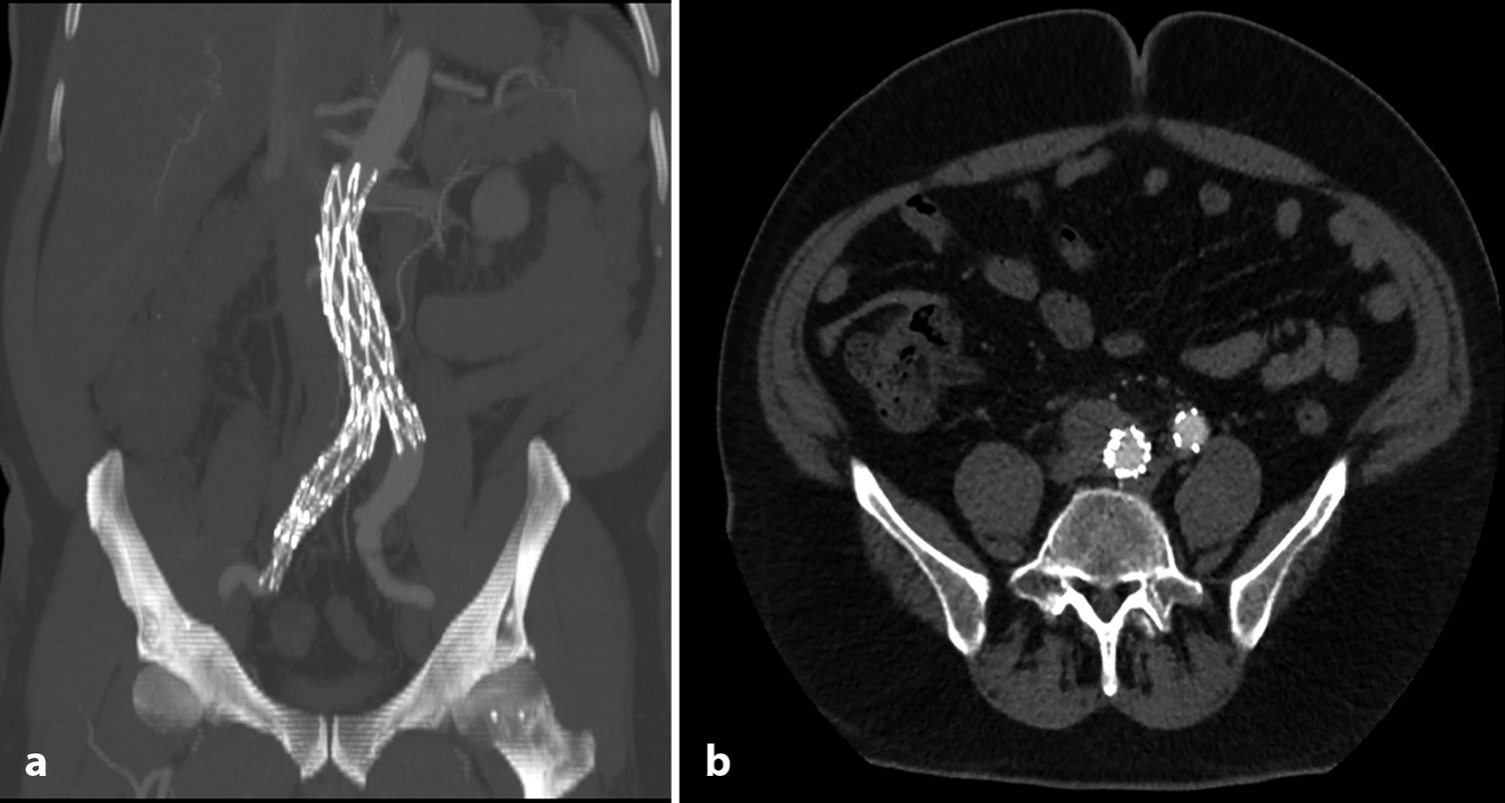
CT surveillance scan performed 3 months after treatment shows the position of the endograft in the aorta and the right common iIiac artery (a). Arterial phase axial image demonstrates complete resolution of inflammatory changes and significant reduction in the size of the aneurysm sac to 3.7 × 2.5 cm (b).
